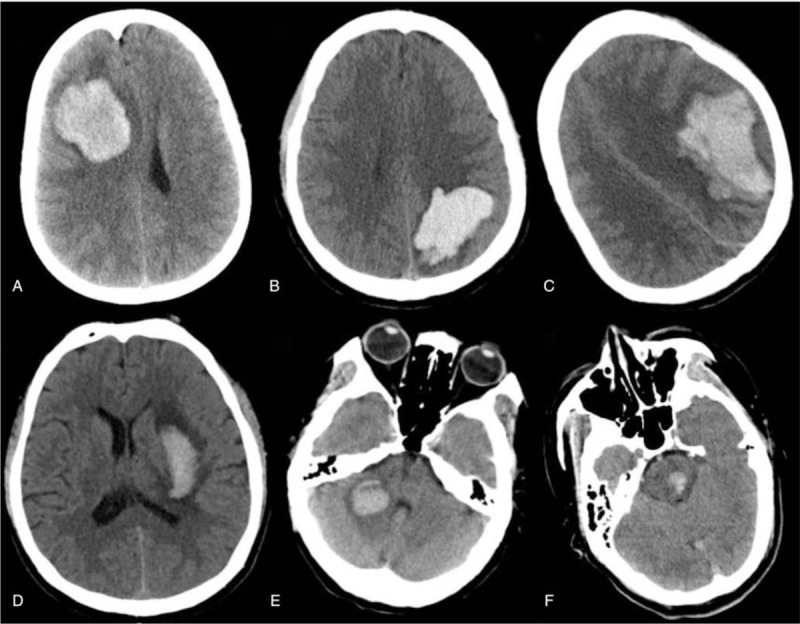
CT images came from 6 active lung patients without conventional vascular risk factors for cerebral hemorrhage. Pictures A–F were brain CT axial view, and the locations of cerebral hemorrhage were shown on the right frontal lobe, left parietal lobe, left frontal parietal temporal lobe, left basal ganglia, right cerebellar hemisphere, and brain stem respectively. It was showed that the lobar hemorrhage was greater than that in other parts of the brain from the above pictures. CT = computed tomography.
